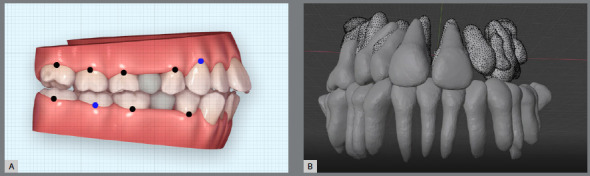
A) Invisalign ClinCheck. B) Blender derived tooth movement from CBCT.
